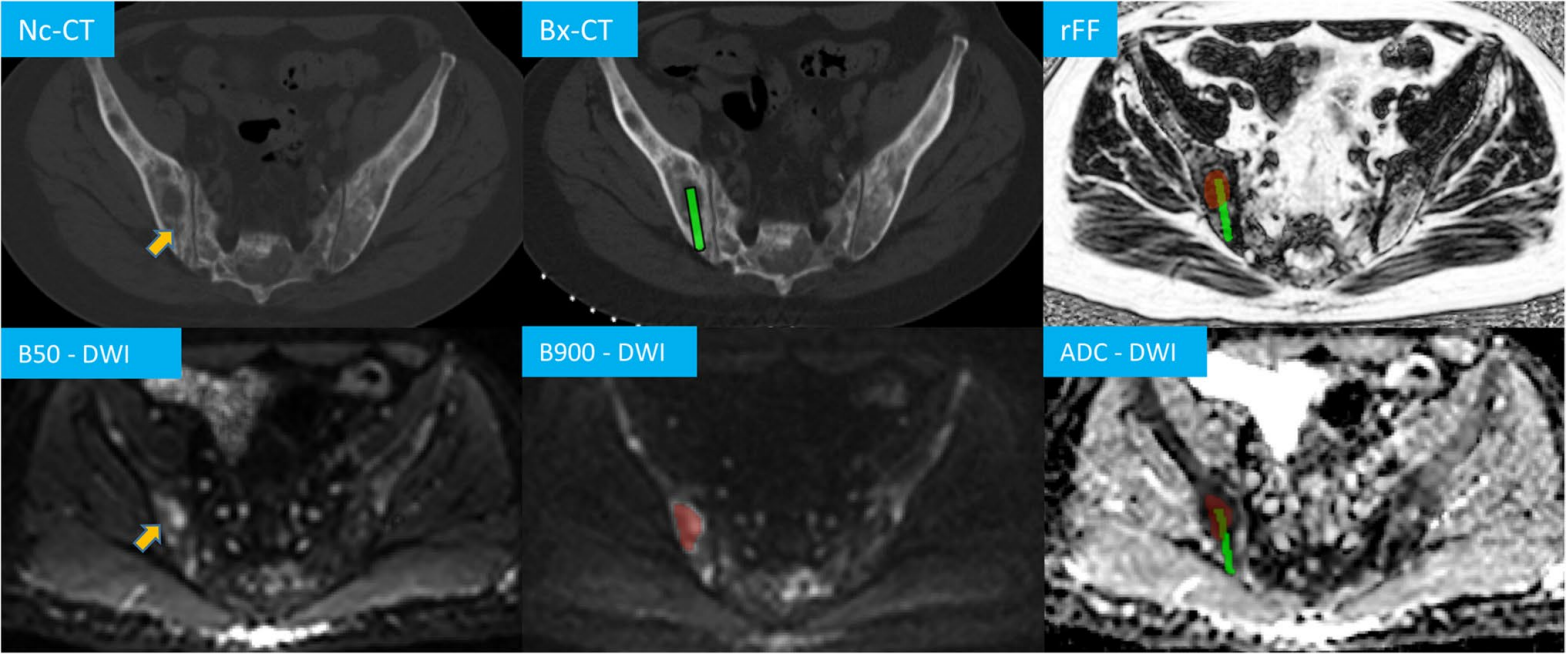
CT and multiparametric MRI of a right iliac bone metastasis in a 53-year-old prostate cancer patient. The biopsy tract (upper orange arrow) on the non-contrast (Nc) CT performed after the biopsy was delineated (green) and transferred onto the planning biopsy CT (Bx-CT), relative fat fraction (rFF), diffusion-weighted (DWI) b900 and ADC images. It intersects the delineated metastasis (light red, orange arrow on b50 image)
